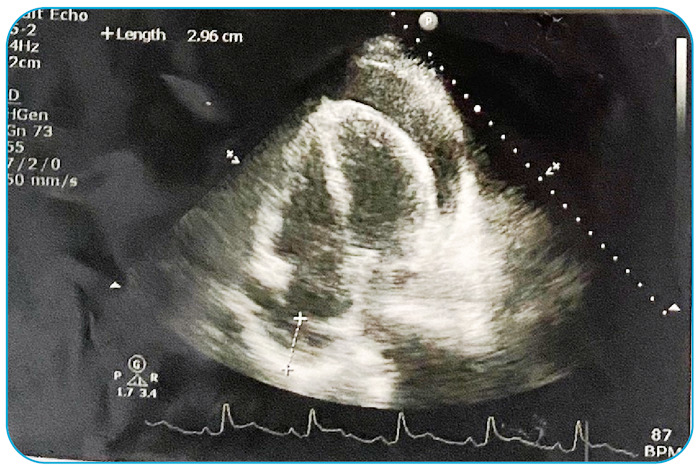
Echocardiography showing pericardial effusion and tamponade signs.
